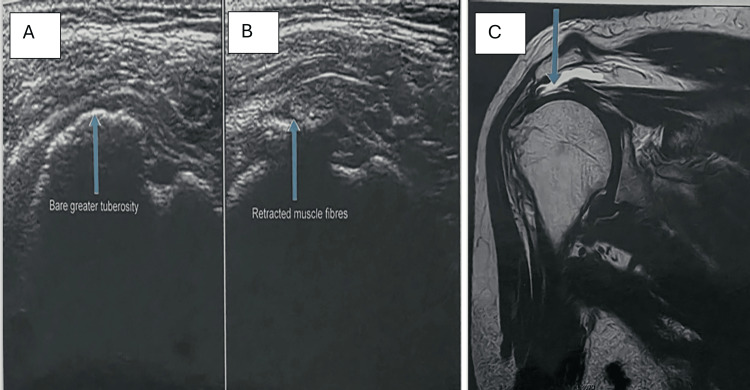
Full-thickness supraspinatus tendon tear on USG and MRI. (A-B) USG showing discontinuity and retraction of supraspinatus tendon fibers with exposure of the greater tuberosity ("bare tuberosity sign"). (C) Coronal T2-weighted MRI image showing nonvisualization of the supraspinatus tendon with proximal tendon retraction (arrow) and mild subacromial bursal effusion USG, ultrasonography; MRI, magnetic resonance imaging
